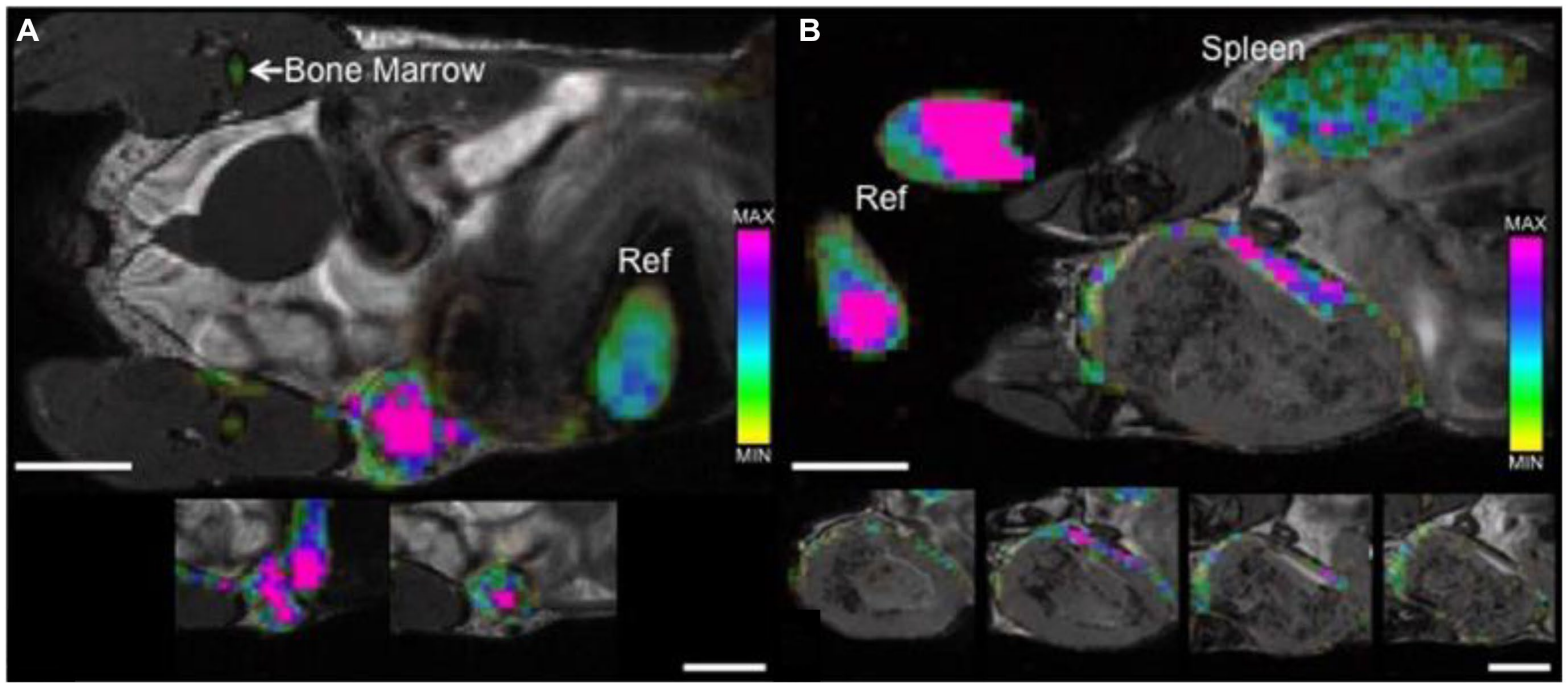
Tracking tumor-associated macrophages by direct labeling with ^19^F contrast agent. bSSFP images of mammary fat pad tumors acquired 48 h post PFC administration. (A) 4 days post 4 T1 cancer cell injection: ^19^F signal is detected throughout the entire tumor with a higher density visualized within the center of the tumor (pink) when compared to the periphery. (B) 3 weeks post 4 T1 cancer cell injection: ^19^F signal is detected, heterogeneous in density, only along the periphery of the tumor. Cropped images that are placed below each of the main images show the adjacent image slices (1 mm) containing ^19^F signal. The color bar demonstrates range of ^19^F spins, ^19^F signal is also detected in reference tubes (Ref), bone marrow and spleen. Scale bars represent 5 mm. [Reproduced from *Sci Rep*, Makela AV et al. “Quantifying tumor associated macrophages in breast cancer: a comparison of iron and fluorine-based MRI cell tracking,” vol. 7, p. 42109, 2017, under a CC BY 4.0 license http://creativecommons.org/licenses/by/4.0/].
